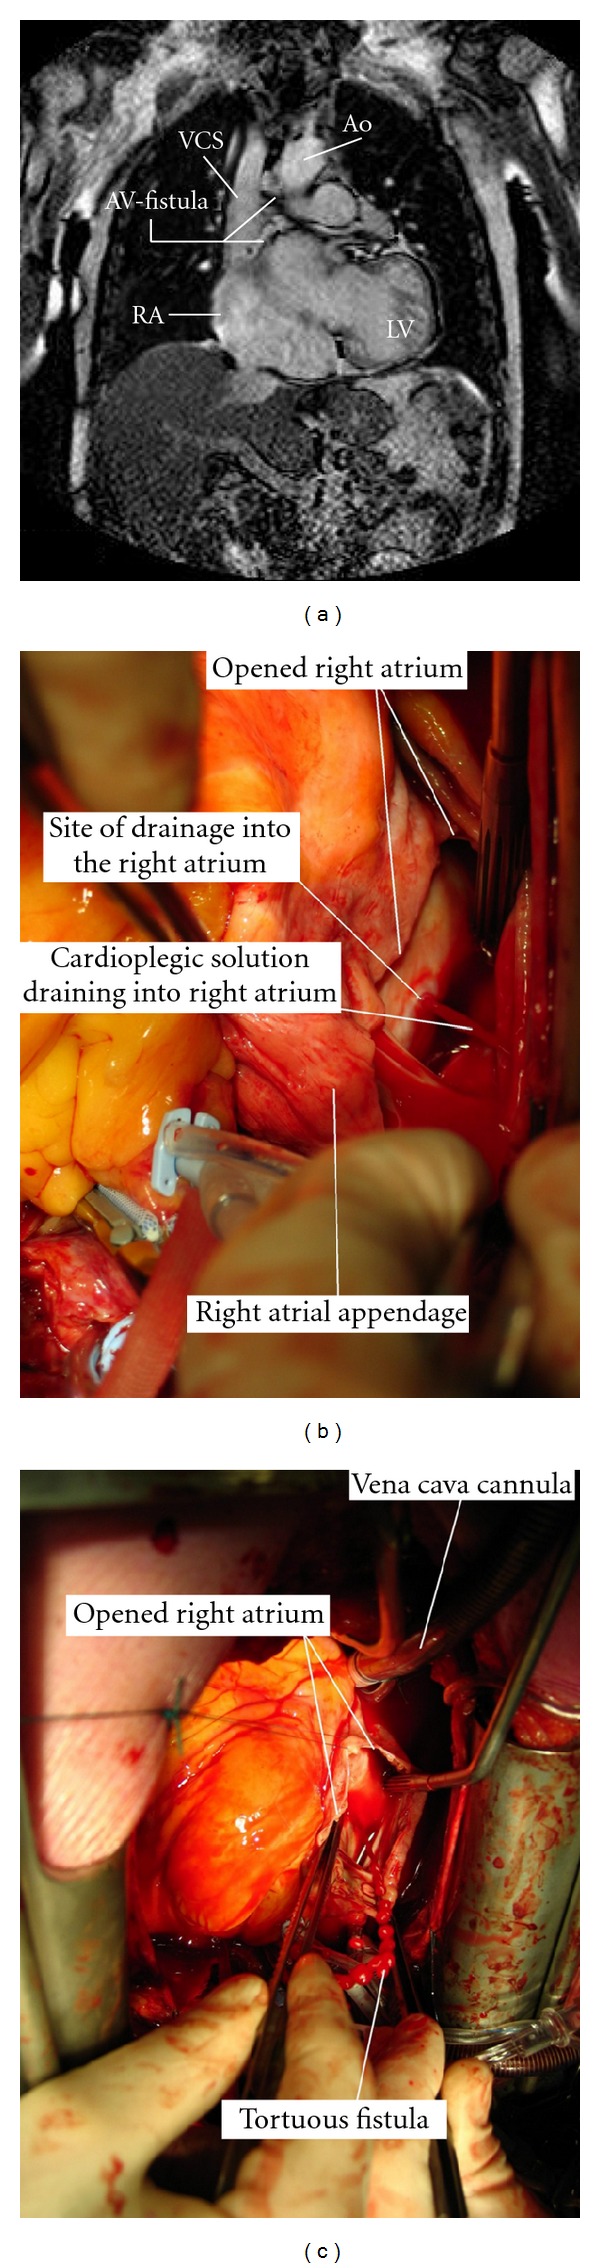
(a) A MRI image showing the arteri-venous malformation originating from the left coronary sinus and draining into the right atrium. Ao = aorta. VCS = vena cava superior. AV = arterio-venous. RA = right atrium. LV = left ventricle. (b) Intra operative image with the site of drainage into the right atrium. (c) Intra-operative image showing the very tortuous arterio-venous fistula.
